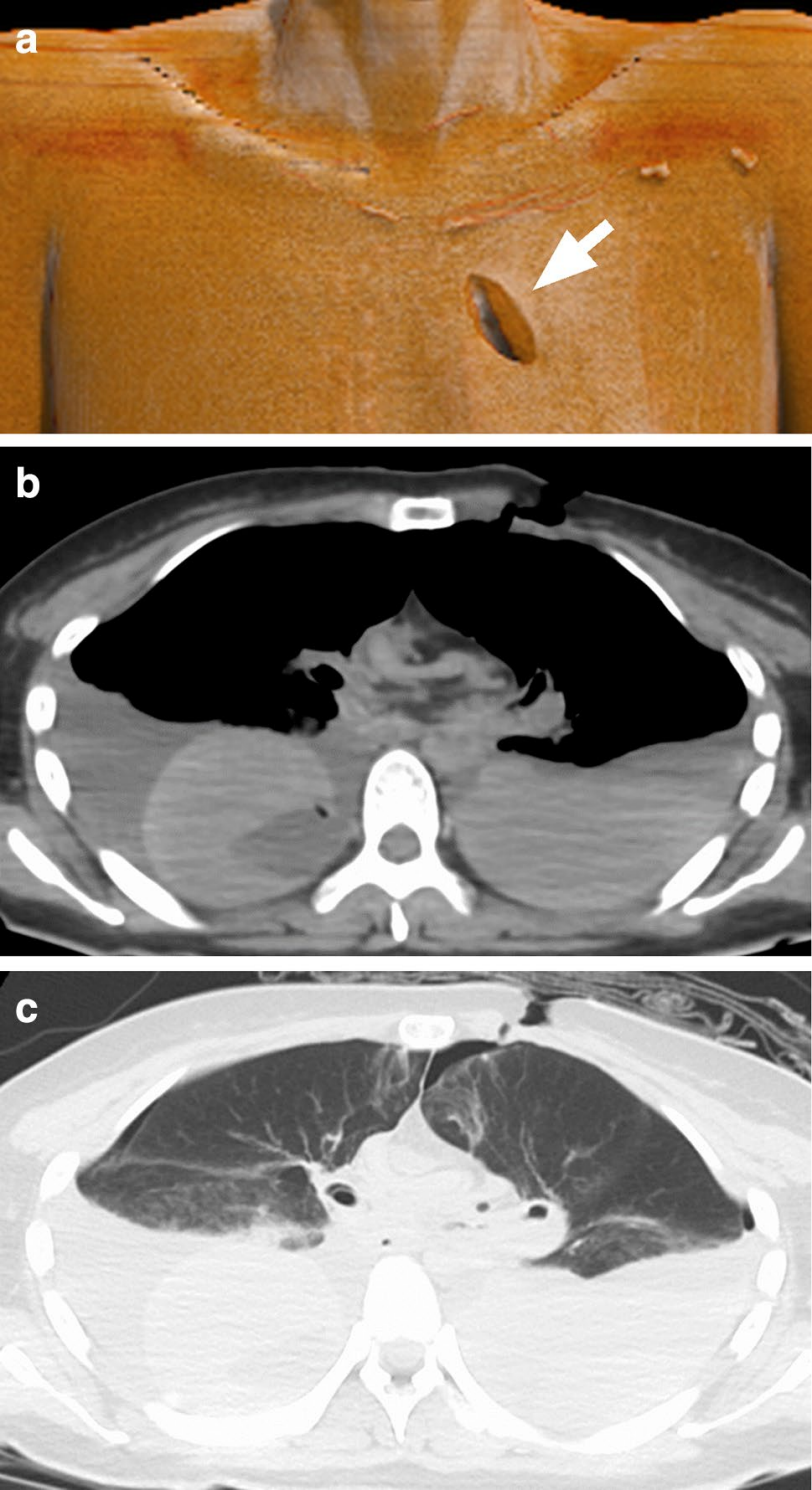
A 49-year-old woman killed with a knife. ((a) 3D CT GIR image, (b) axial CT image (soft kernel), (c) axial CT image (lung kernel)). (a) 3D CT GIR image showing a stab wound at the left anterior thorax (arrow). (b,c) The axial CT images show bilateral hemopneumothorax suggesting that the knife stabbed into the left anterior thorax penetrated the mediastinum and reached the right lung.
